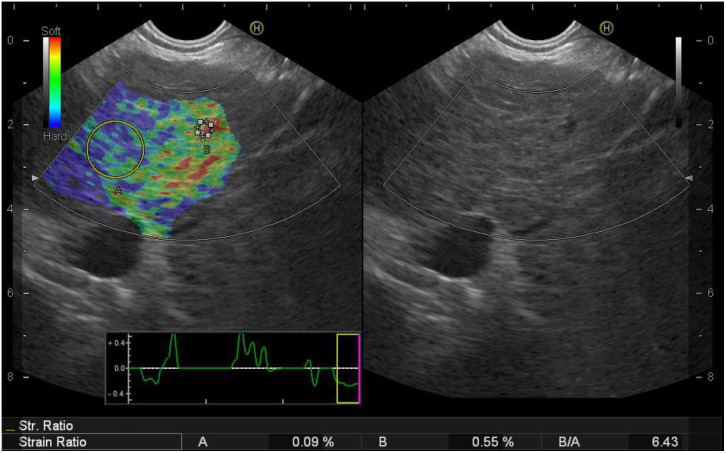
Strain measurement using endoscopic ultrasound strain elastography.
